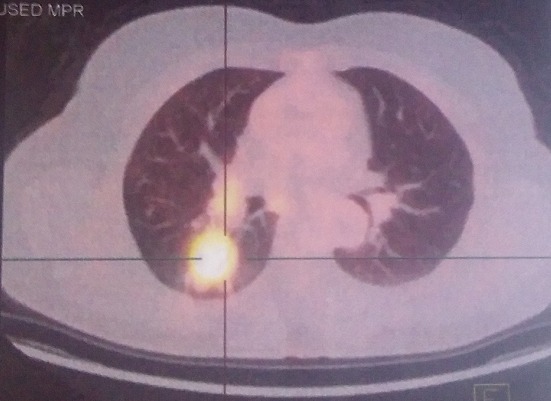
La masse pulmonaire droite sur un foyer hyper métabolique (SUV max 7,54; Volume métabolique 17,68 cm3) avec un hyper métabolisme hilaire droit (SUV max 5,56) et une adénopathie hyper métabolique sous carinaire (SUV max 4,35)
